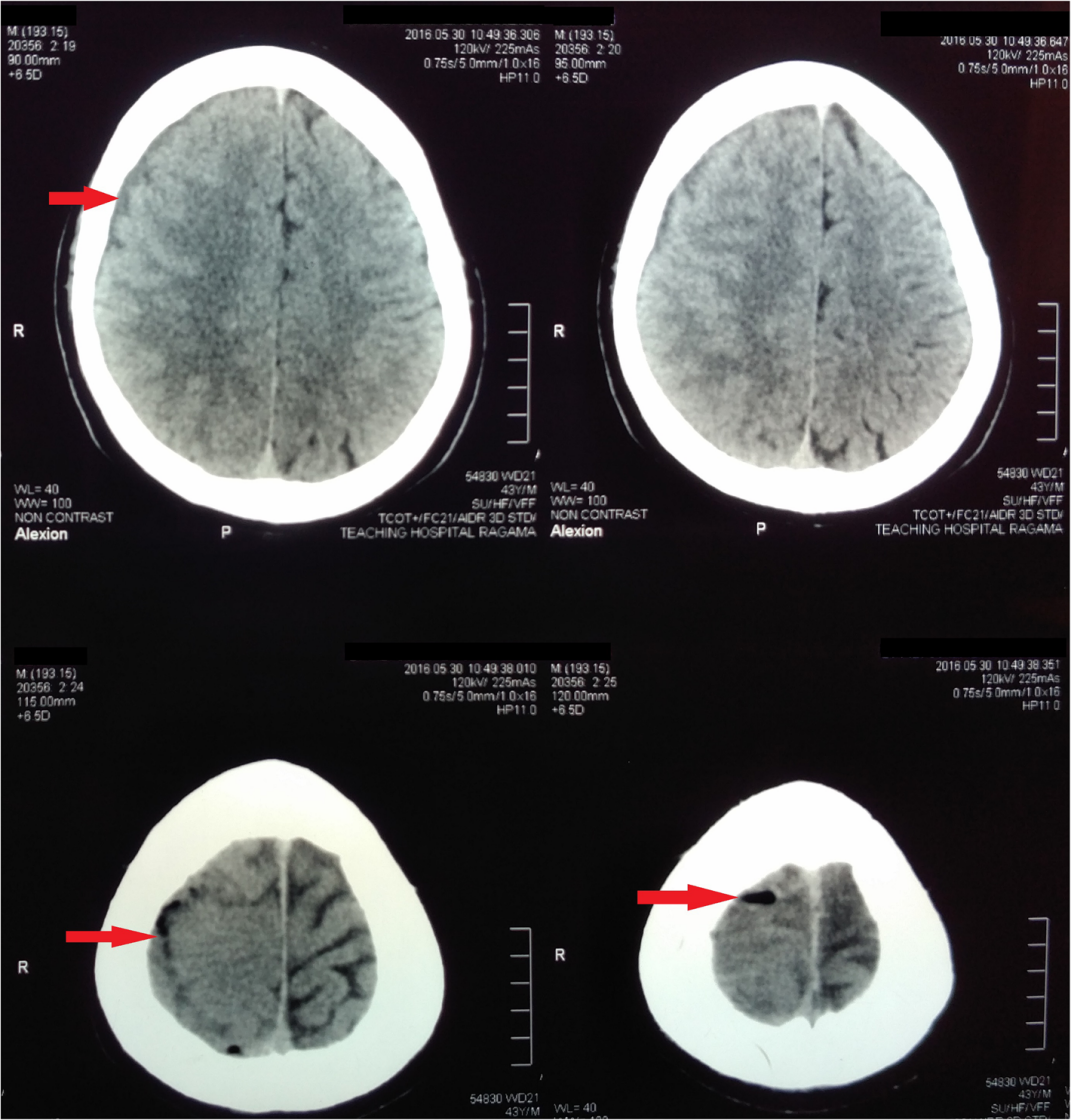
Pre-treatment non-contrast computed tomography of brain showing a right fronto-parietal subdural collection with gas locules
